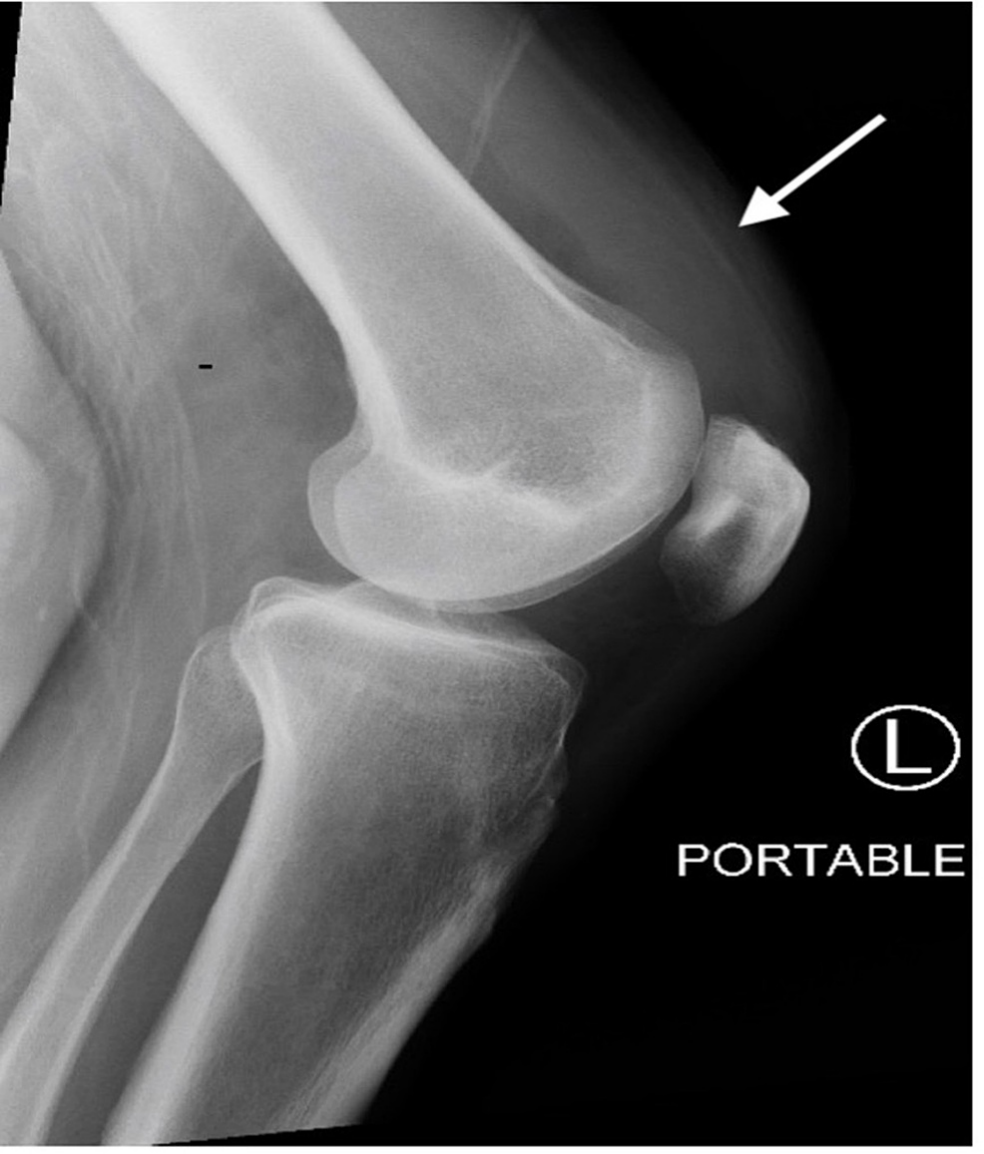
Lateral left knee X-ray revealing significant effusion (arrow), remarkable for septic arthritis
